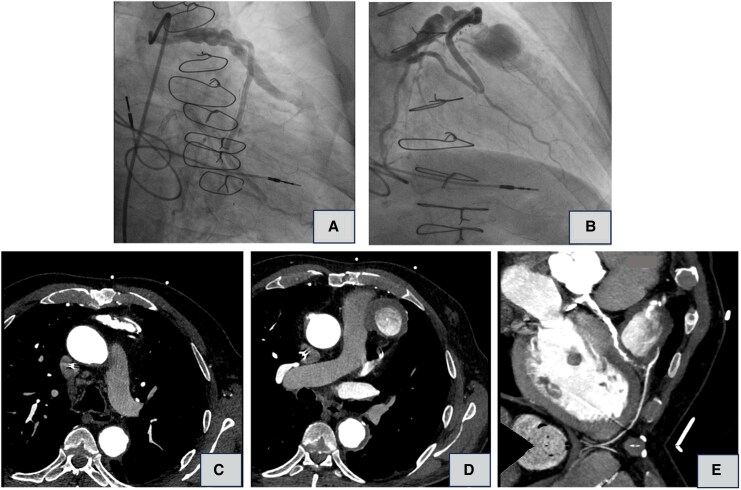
(*A*) Aneurysmatic dilatation of the saphenous vein graft (SVG) to the diagonal branch as presented in the coronary angiography performed 3 years ago. (*B*) Substantial extravasation of contrast medium from the SVG into a giant circular aneurysmatic formation as shown in the new coronary angiography (corresponding dynamic sequence available in Supplementary material online, *Video S1*) (*C*—*E*) Computed tomography demonstrating a 43 × 47mm pseudoaneurysm in front of the anterior left ventricular wall, adjacent to the left anterior descending artery and the left internal mammary artery, perfused by the aneurysmatic SVG (corresponding imaging sequence available in Supplementary material online, *Video S2*).
